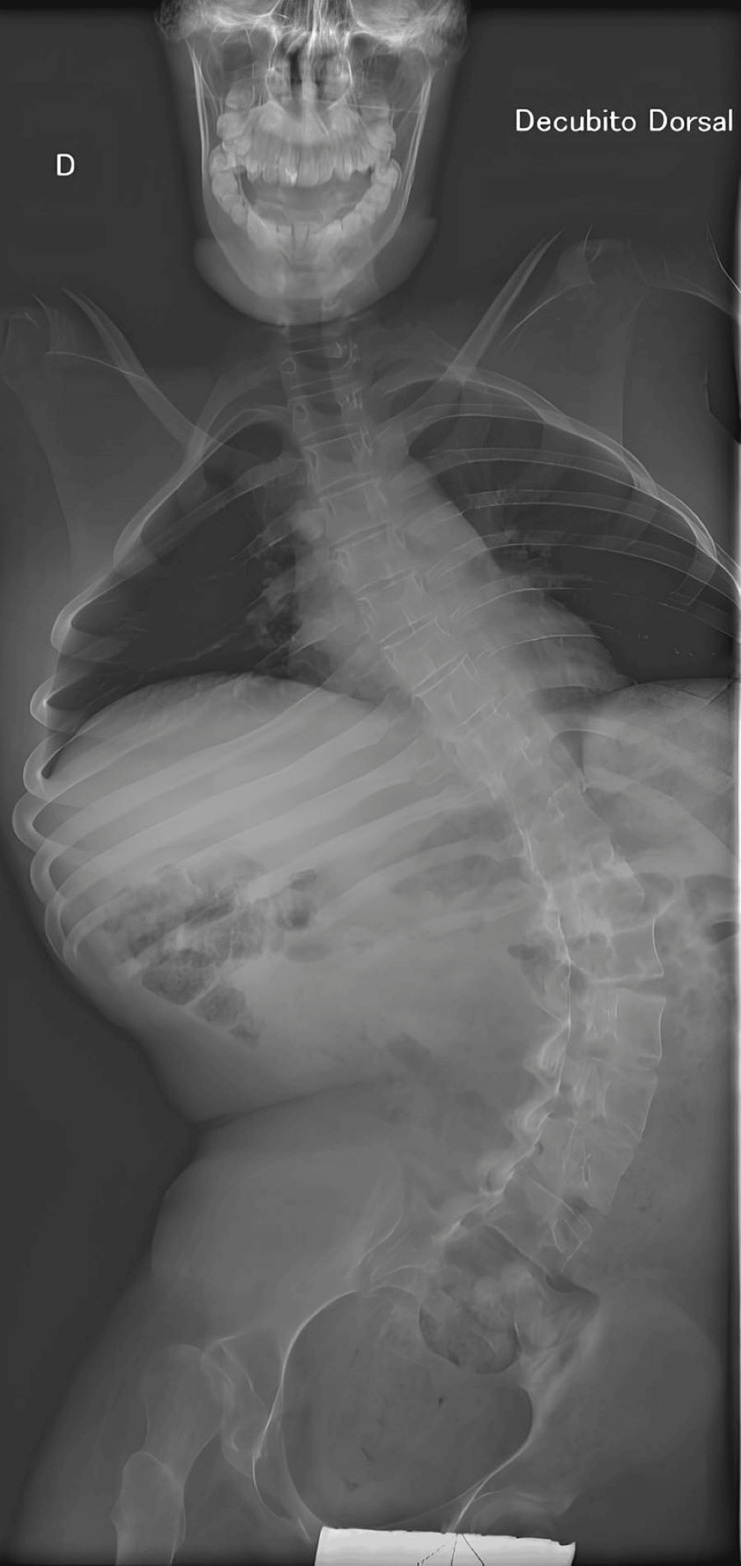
Pre-operative X-ray Moderate thoracolumbar scoliosis (Cobb angle 35.6º); Decubito dorsal - supine
